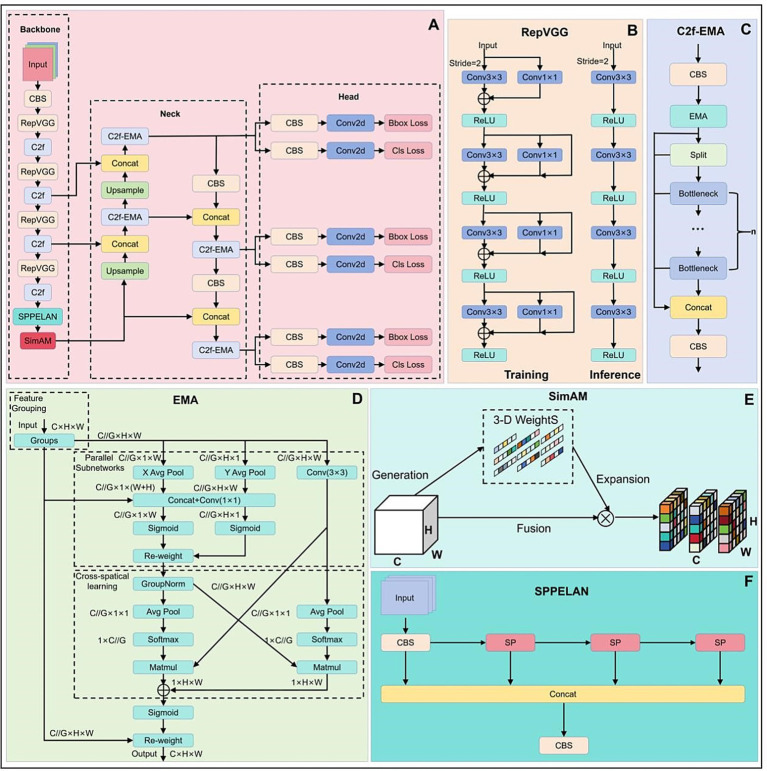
The improved YOLOv8 network model and the specific network structure of the improved parts. We modified and replaced some modules of the original YOLOv8 network model to make it more suitable for MRI image recognition (A). Firstly, in the backbone network, the CBS convolution modules of layers 1, 3, 5, and 7 were replaced with RepVGG modules (B) to enhance the training accuracy and inference speed of Alzheimer’s disease MRI medical images; Secondly, the original spatial pyramid pooling layer SPPF is replaced with SPPELAN (F), which enhances the recognition ability of Alzheimer’s disease images by fusing local and global features at multiple scales; Then, SimAM attention mechanism (E) was added at the end of the backbone network, enabling the network to better focus on channel attention and achieve feature focus detection of more important and informative features in Alzheimer’s disease images; Finally, some C2f modules in the neck network FPN-PAN were replaced with C2f_EMA modules (C), By adding EMA attention mechanism (D) to the C2f module, the input feature weights in the C2f module were dynamically adjusted, enhancing the model’s generalization ability and recognition accuracy for Alzheimer’s disease MRI medical images.
